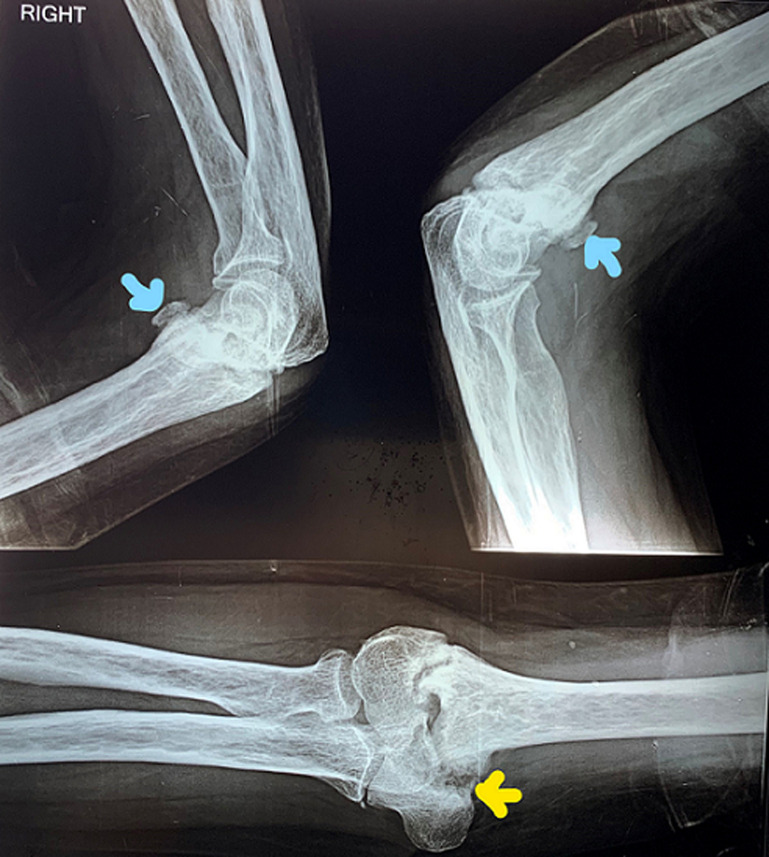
X-ray showing right elbow in lateral and antero-posterior (AP) views (blue arrows show bone chip and yellow arrow shows unhealed supracondylar fracture of humerus)
